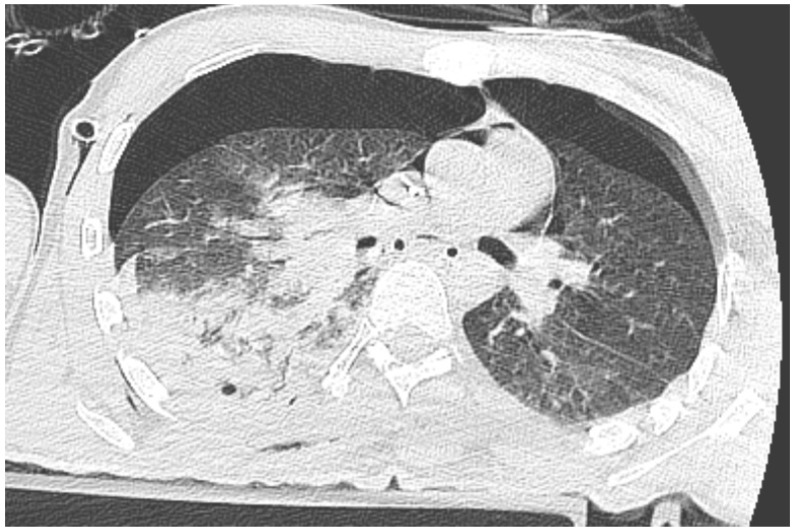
CT scan of pneumothorax and pneumocardium after pericardial decompression of the pneumopericardium.
